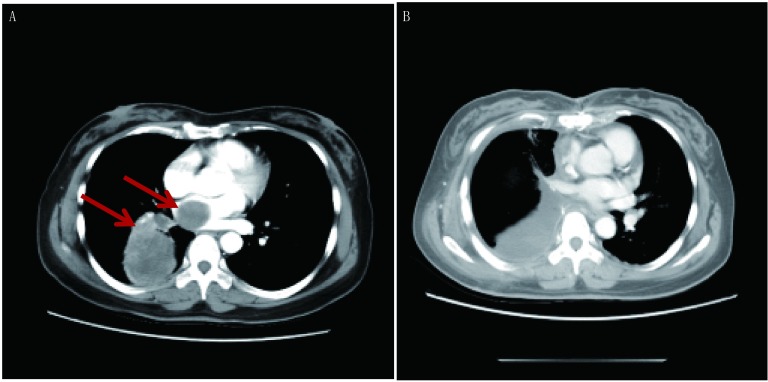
右肺平滑肌肉瘤累及左心房（箭头示），术前及术后早期对比（肿瘤全部切除）。A：术前；B：术后3周。 Right lung leiomy-osarcoma involving the left atrium (Arrow), preoperative and postoperative CT scan (The tumors were removed completely). A: preoperative; B: 3 weeks after operation. CT: computed tomography.
